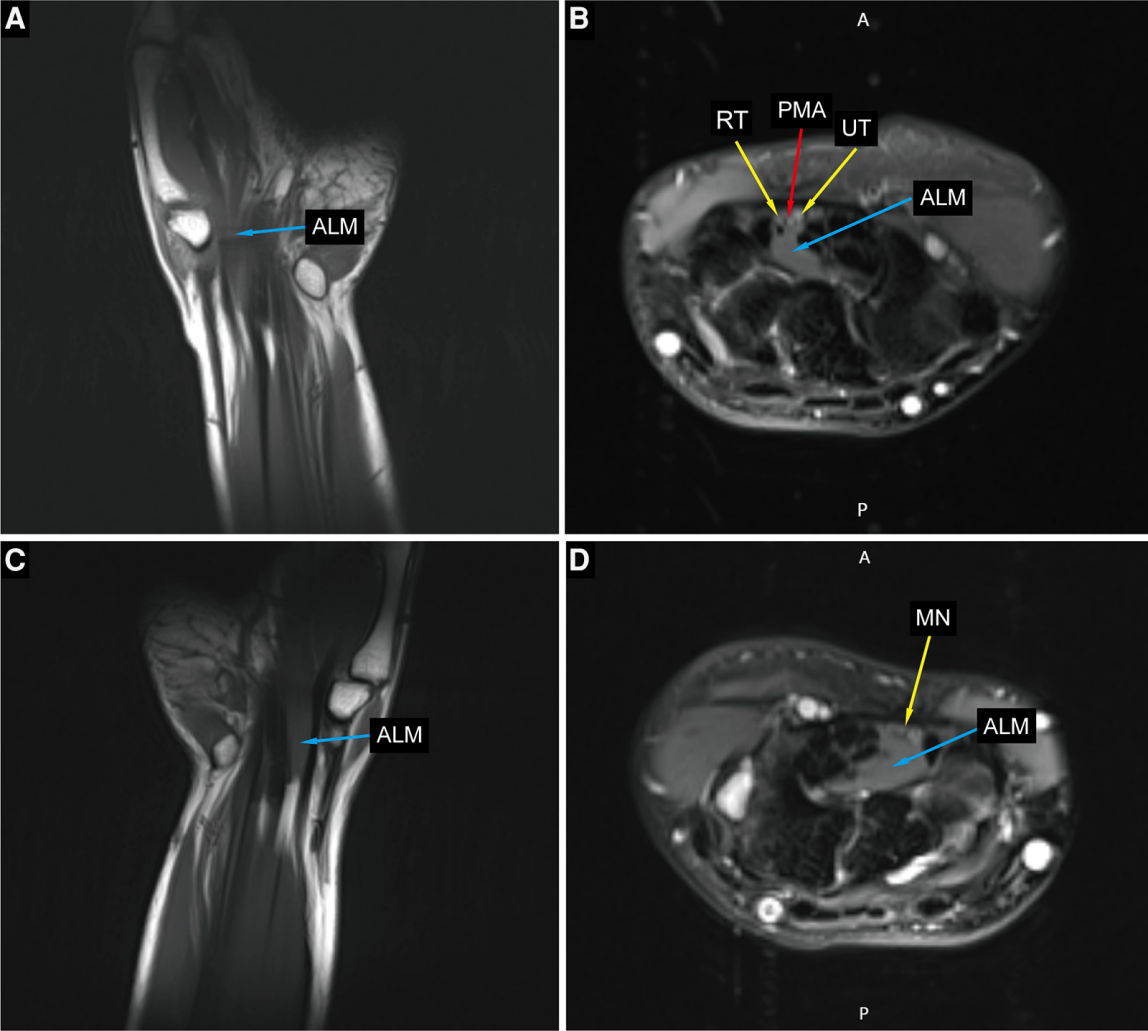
Preoperative MRI imaging of the bilateral hands and wrists. (A,C) Coronal sections of the left and right hand show an anomalous lumbrical muscle in carpal tunnel. (B) Axial section at left hamate hook level showing anomalous lumbrical muscle, bifid median nerve with persistent median artery. (D) Axial section at right hamate hook level showing anomalous lumbrical muscle and compressed median nerve. ALM, anomalous lumbrical muscle; RT, radial trunk of the median nerve; UT, ulnar trunk of the median nerve; PMA, persistant median artery; MN, median nerve.
